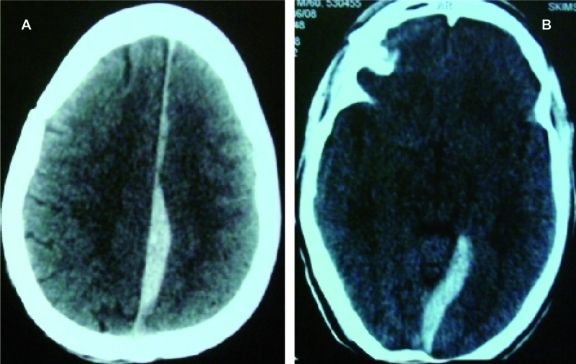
Axial CT scan after head injury following road traffic accident revealing interhemispheric acute subdural hematoma.
